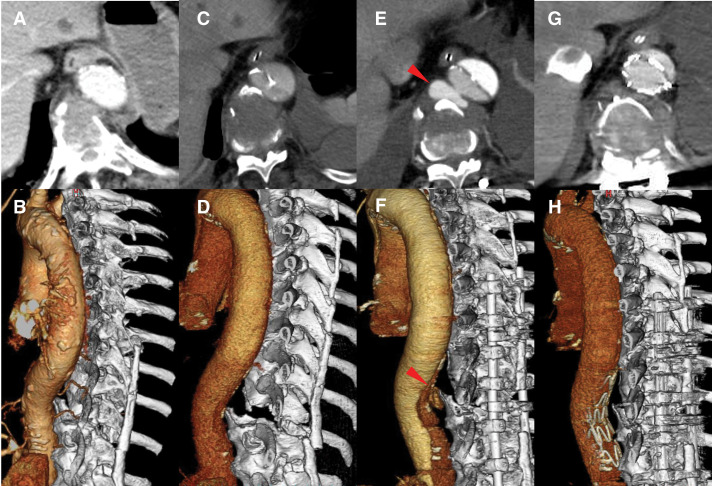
Time course of a delayed thoracic aortic pseudoaneurysm associated with a thoracic vertebral fracture. (A) and (B) CT reveals the 10th thoracic vertebra near the descending aorta on admission. (C) and (D) CT on day 19 reveals the 10th thoracic vertebra to be in contact with the descending aorta; however, no pseudoaneurysm is observed. (E) and (F) CT shows a new pseudoaneurysm in contact with the 10th thoracic vertebra on day 34 (arrows). (G) and (H) CT on day 38 confirms resolution of the pseudoaneurysm.
